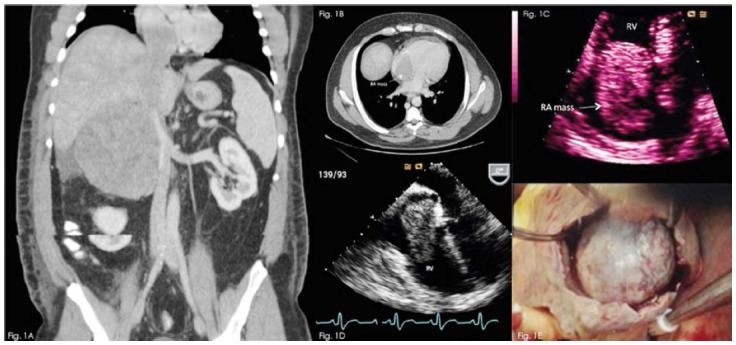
Fig.1A: Computed tomography (coronal view) shows a large, heterogeneous and lobulated mass arising from the right adrenal gland, measuring 10 cm x 12 cm x 22 cm, which extends into the inferior vena cava and right atrium. Fig.1B: Computed tomography (axial view) reveals a filling defect caused by the tumor which occupies the entire right atrium to the level of tricuspid valve. RA = right atrium Fig.1C: Transthoracic echocardiogram (apical 4 chamber zoomed-in view) of the right atrial mass prolapsing through the tricuspid valve into the right ventricle. RA = right atrium; RV = right ventricle. Fig.1D: Transesophageal echocardiogram (mid esophageal 4 chamber view) showing the right atrial mass prolapsing through the tricuspid valve in to the right ventricle. RA = right atrium; RV = right ventricle. Fig.1E: Intraoperative photograph of the right atrial mass.
